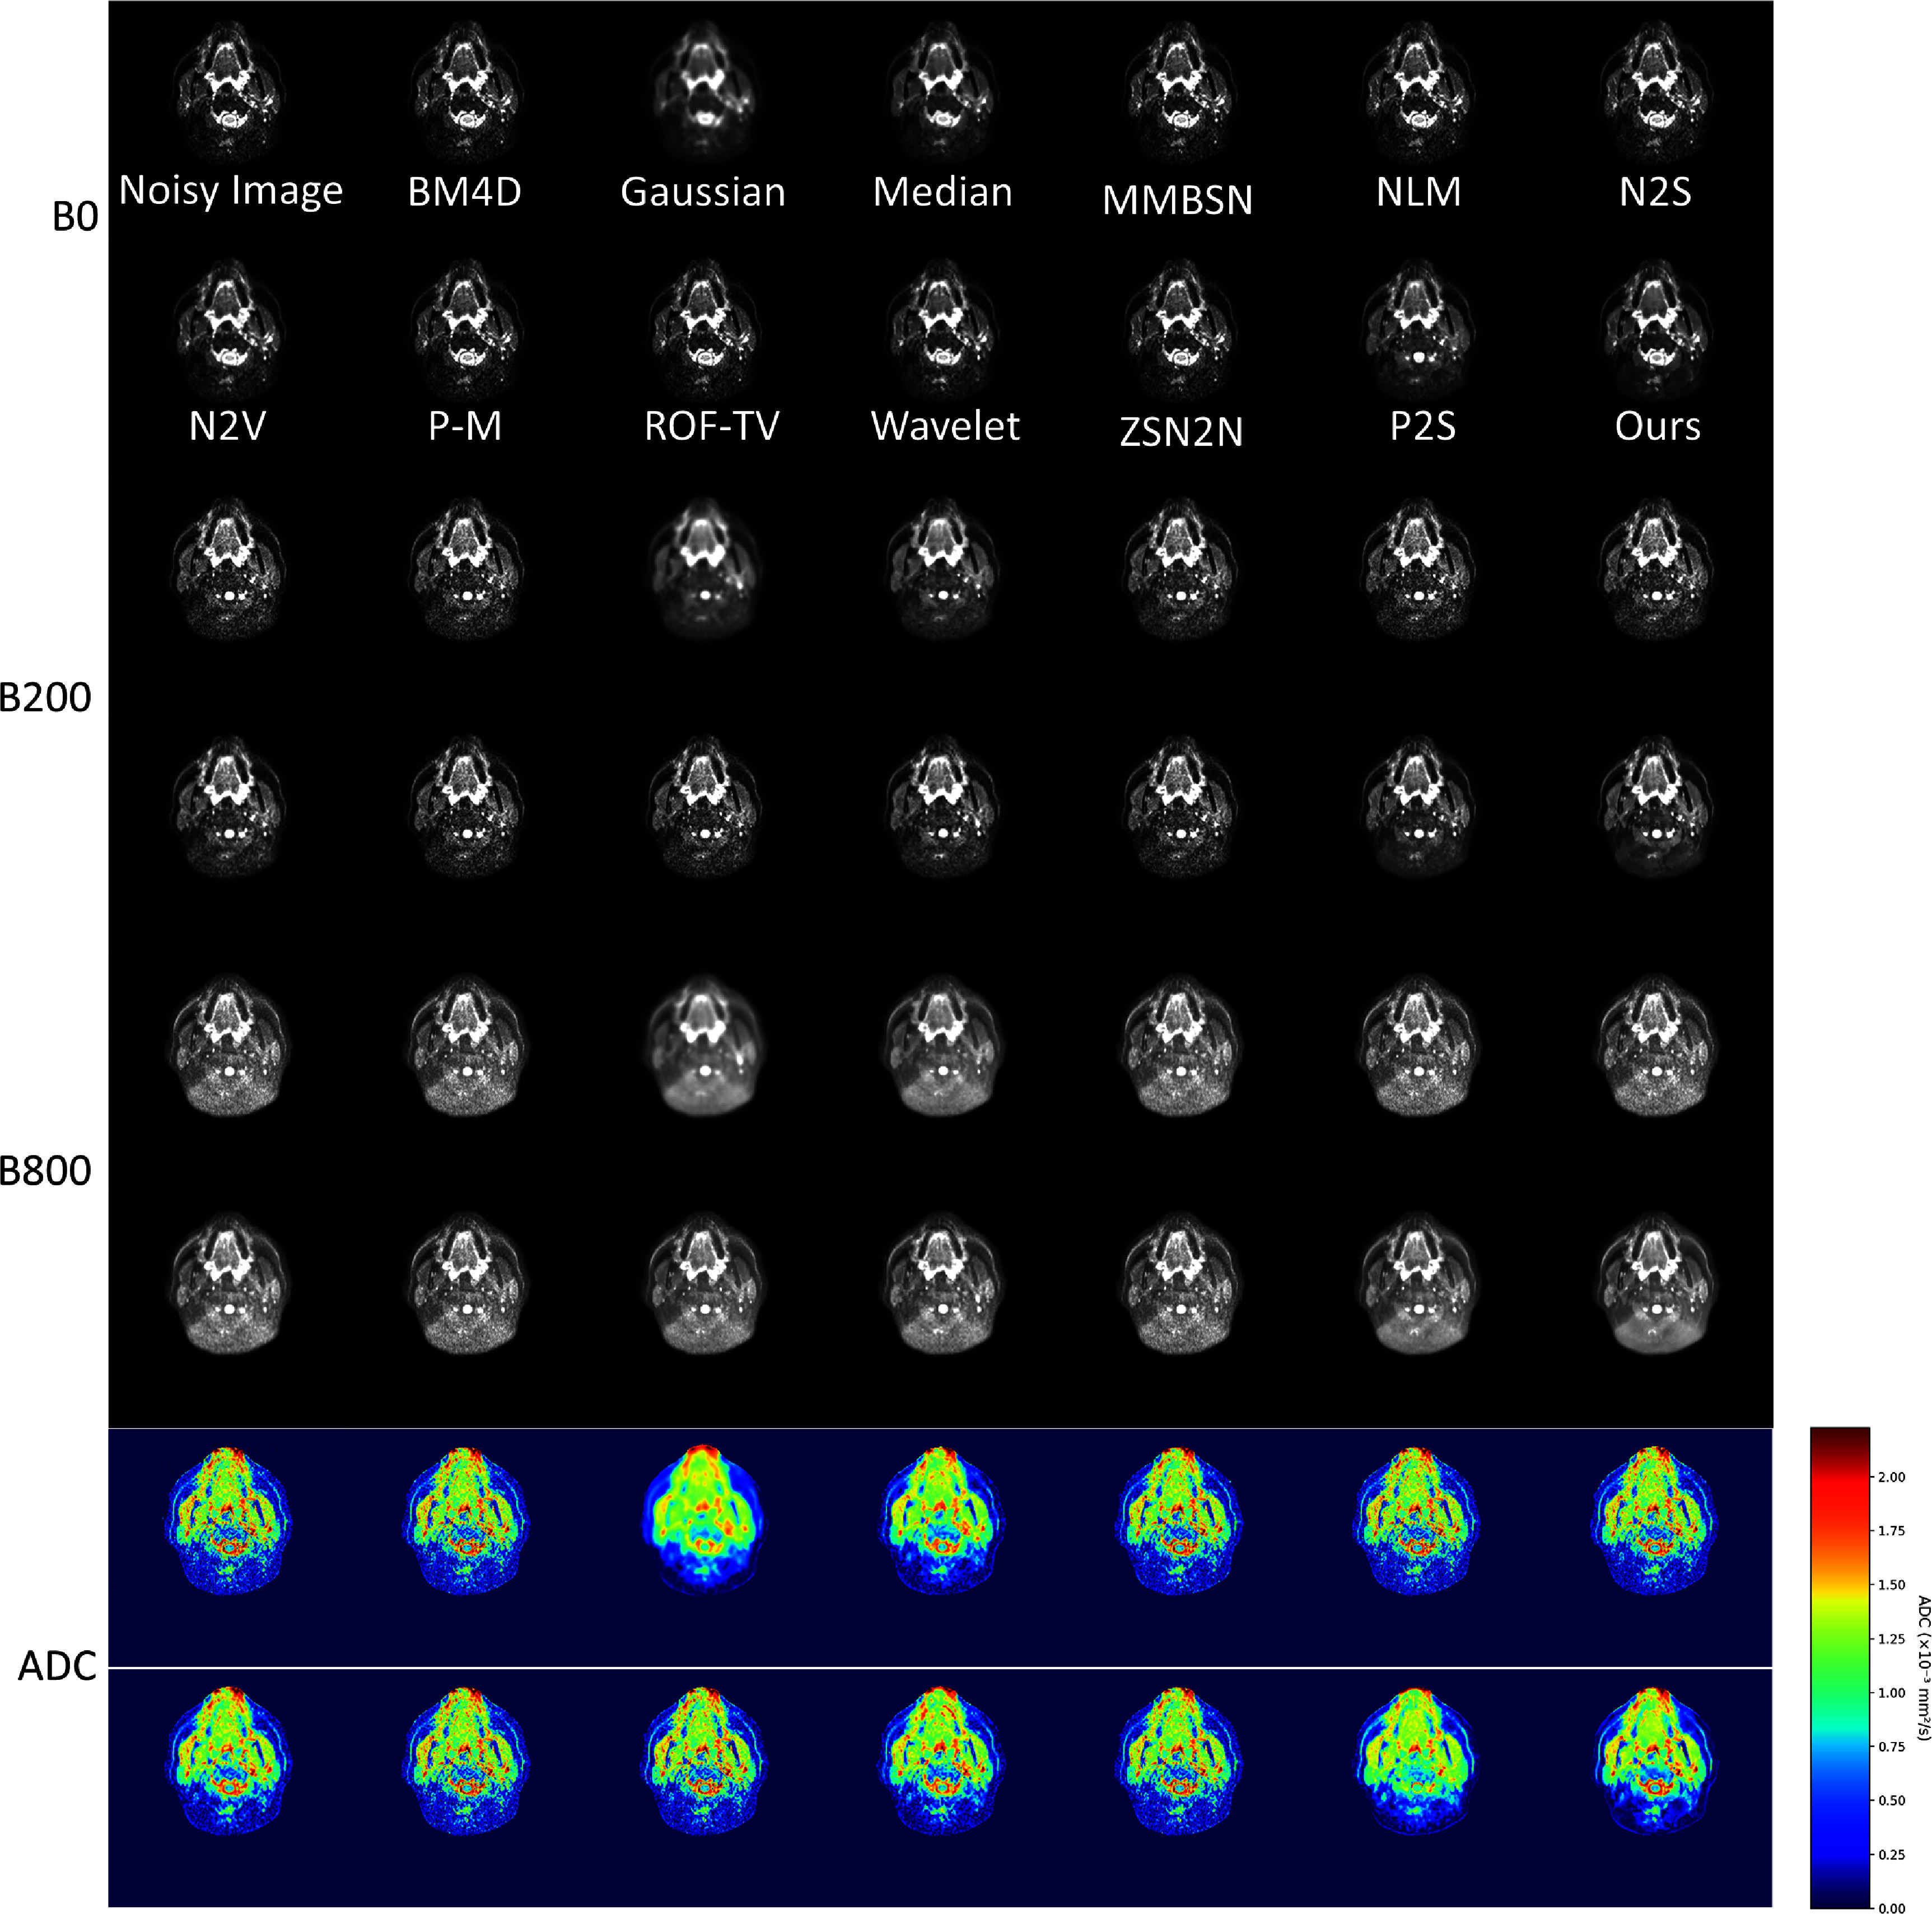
Comparison of head and neck DWI denoising results. The complex structures in head and neck regions pose unique challenges for denoising algorithms, and BL-INR maintains excellent performance.
